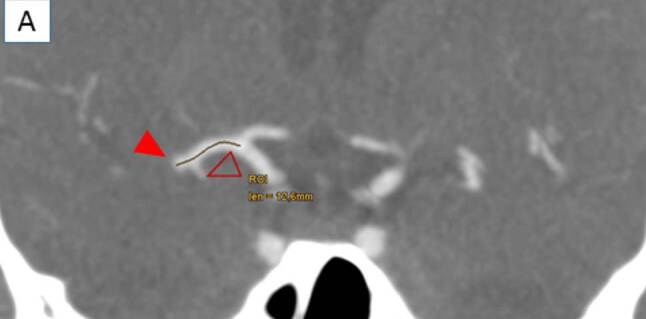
Depiction of the measurement of the distance to thrombus (DT) from the middle of the T-Bifurcation of the internal carotid artery (empty arrow) to the cessation of contrast medium as the proximal end of the thrombus (filled arrow) based on a CT-angiography (12.6 mm in this example)
